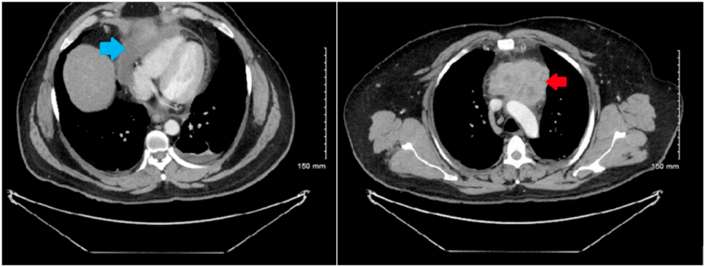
CT Chest with contrast demonstrating a heart that is normal in size with a right pericardial effusion measuring 98 × 29 mm (blue arrow). Large irregular inhomogeneous retrosternal/superior mass measuring 7.8 × 5.7 × 5.8 cm (red arrow). (For interpretation of the references to colour in this figure legend, the reader is referred to the Web version of this article.)
